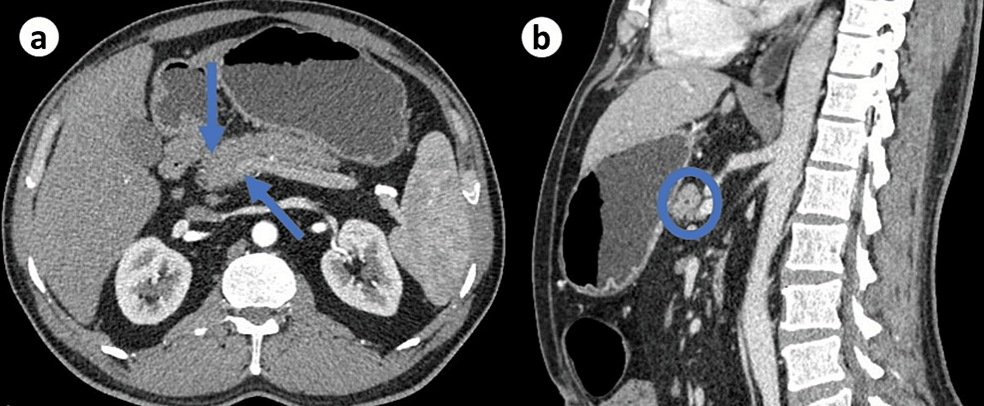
CT abdomen showing pancreatic head enlargement with irregular borders (a) and concentric thickening of the pancreatic duct (b). CT: computed tomography.
